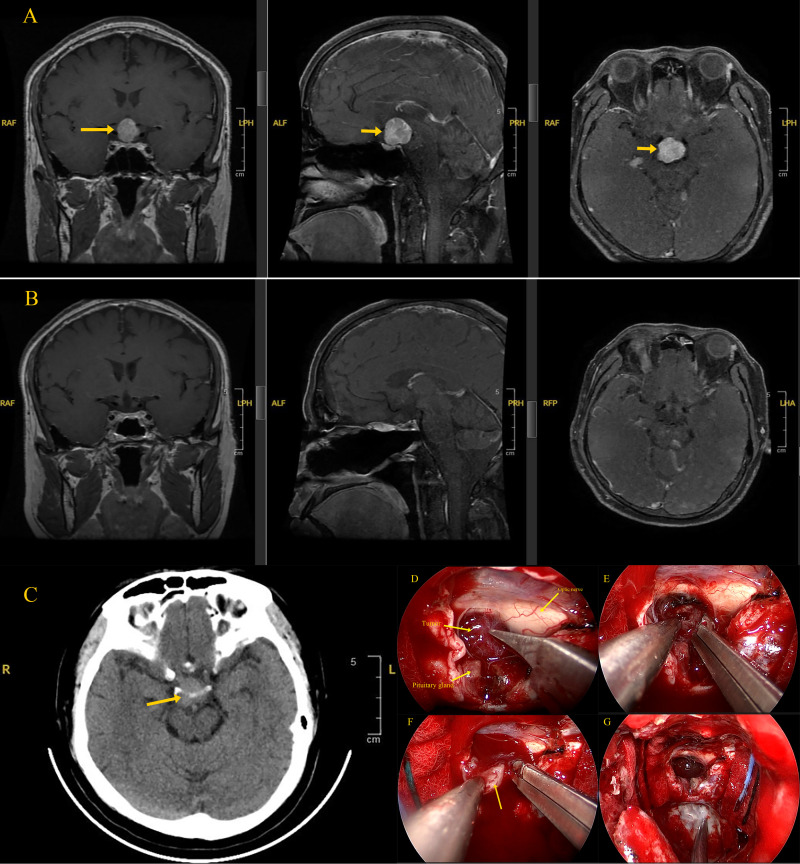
(A) preoperative sagittal, coronal, and vertical-axis MRI of the brain showing a tumor in the saddle region; (B) postoperative sagittal, coronal, and vertical-axis MRI of the brain showing tumor removal; (C) Cranial CT showing a space-occupying lesion in the saddle region; (D) The positional relationship between the tumor and surrounding tissues; (E) Intraoperative endoscopic view showing tumor exposure with sparse vascular distribution on its surface and clear boundaries with adjacent tissues; (F) Intraoperative endoscopic view demonstrating extremely rich tumor blood supply, accompanied by significant bleeding during resection; (G) Intraoperative endoscopic images showing complete tumor removal, with no residual tumor tissue observed in the surgical area.
